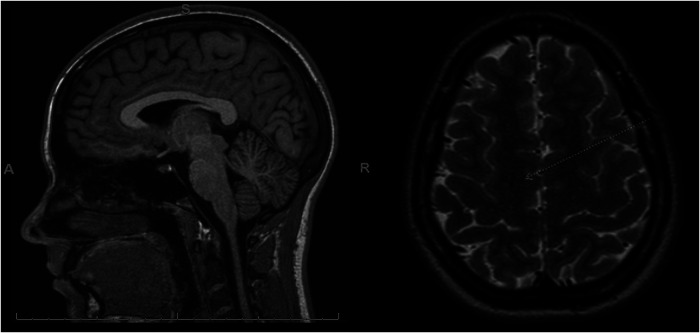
MRI post-concussive brain imaging of the patient. In this case, the patient received an MRI 2 weeks after injury. The MRI revealed an incidental right frontal developmental venous anomaly (white arrow) and was otherwise normal. No pre-concussive MRIs are available for comparison.
